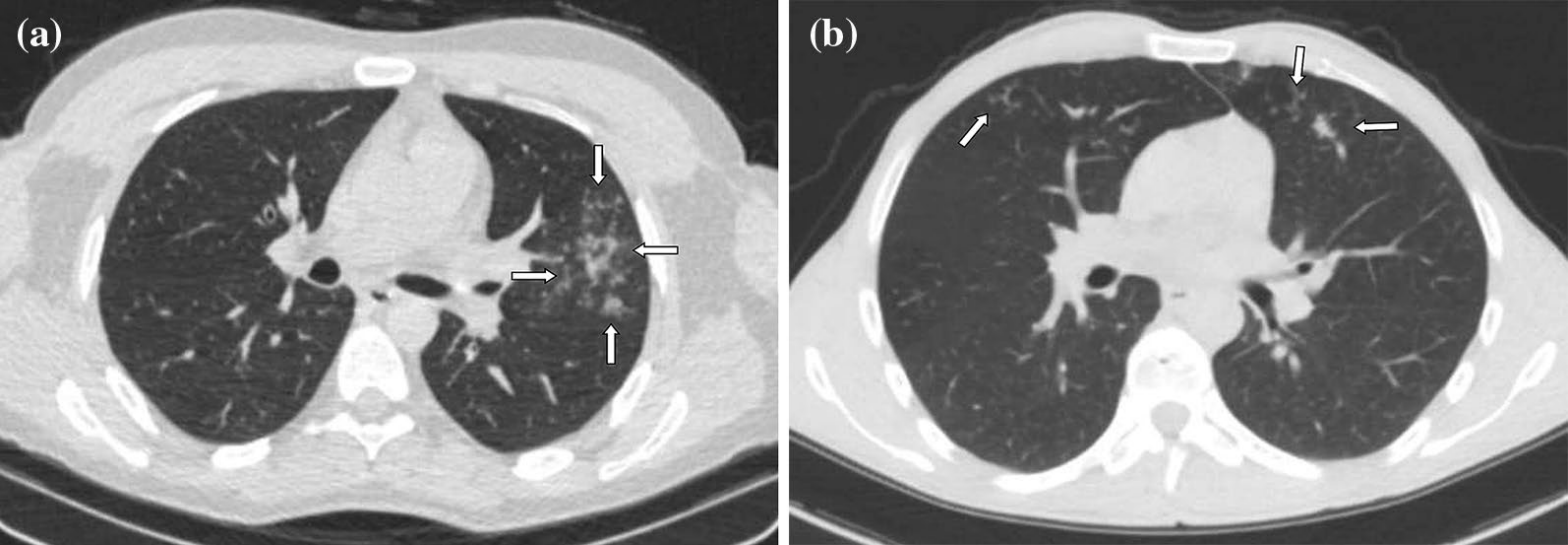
a, b Case examples defined in the non-COVID for the BSTI guidance statement, and in the atypical category for the RSNA expert consensus statement. a An axial chest CT image of a 36-year-old male without COVID-19 shows centrilobular nodules without GGOs in the left upper lobe (arrows). The final diagnosis was bronchial pneumonia. b An axial chest CT image of a 37-year-old male without COVID-19 shows centrilobular nodules and tree-in-bud appearance without GGOs in the right middle and left lingula segment (arrows). The final diagnosis was of lung tuberculosis
